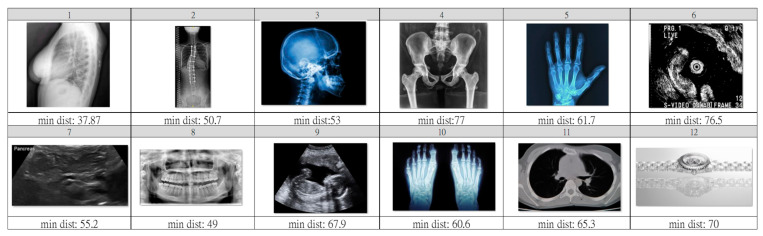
Outlier set and minimum L2 distance to three cluster centers. The number one image, lateral view X-ray, has the shortest similarity distance.
